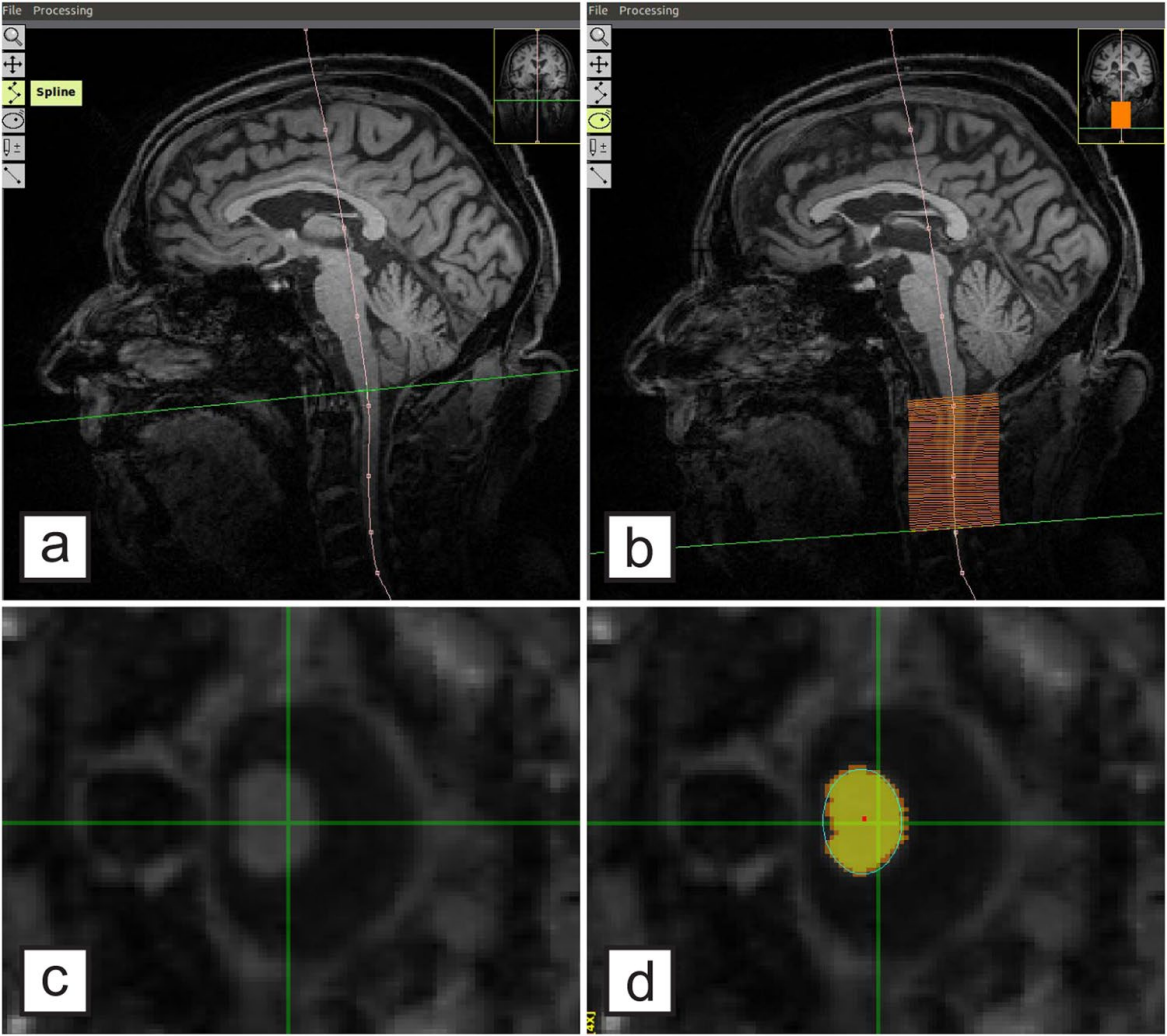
Measurement of the cervical spinal cord cross-sectional area (CSA) applying SpineSeg. (a) demonstrates the cerebral 3T MRI, covering the upper segments of the cervical spinal cord, in a sagittal view. A guiding curve (pink) was placed along the spinal cord in the coronal and sagittal plane; the image is consequently resampled into perpendicular slices. Within the slices from the dens axis until the second intervertebral disk (b), the cervical spinal CSA segmentation was derived using a semi-automated tree pruning approach. Transversal view of the spinal cord is demonstrated before (c) and after (d) segmentation, respectively. (d) Seed in red, segmentation in yellow.
